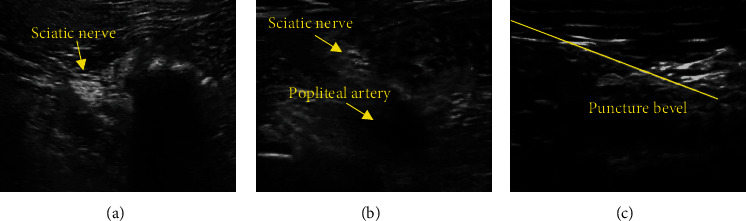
Ultrasound images. (a) Ultrasound display of the sciatic nerve; (b) ultrasound display of the femoral artery; (c) ultrasound-guided tip oblique view. The yellow arrows in the figure indicate sciatic nerve and popliteal artery, and the yellow line is puncture bevel.
